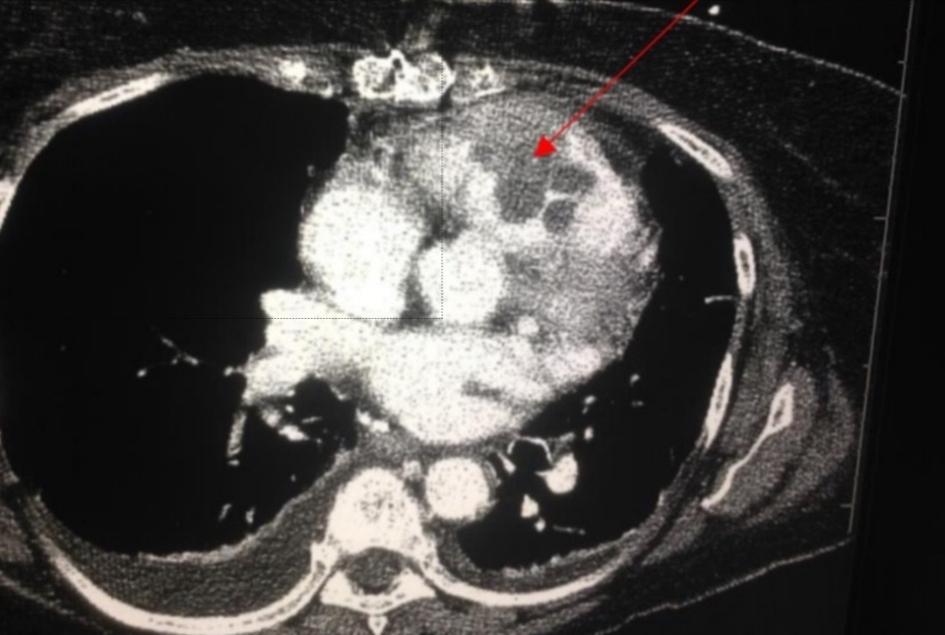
Computed tomography (CT) chest showing a large, multi-lobulated, heterogenous mass in the right ventricle
